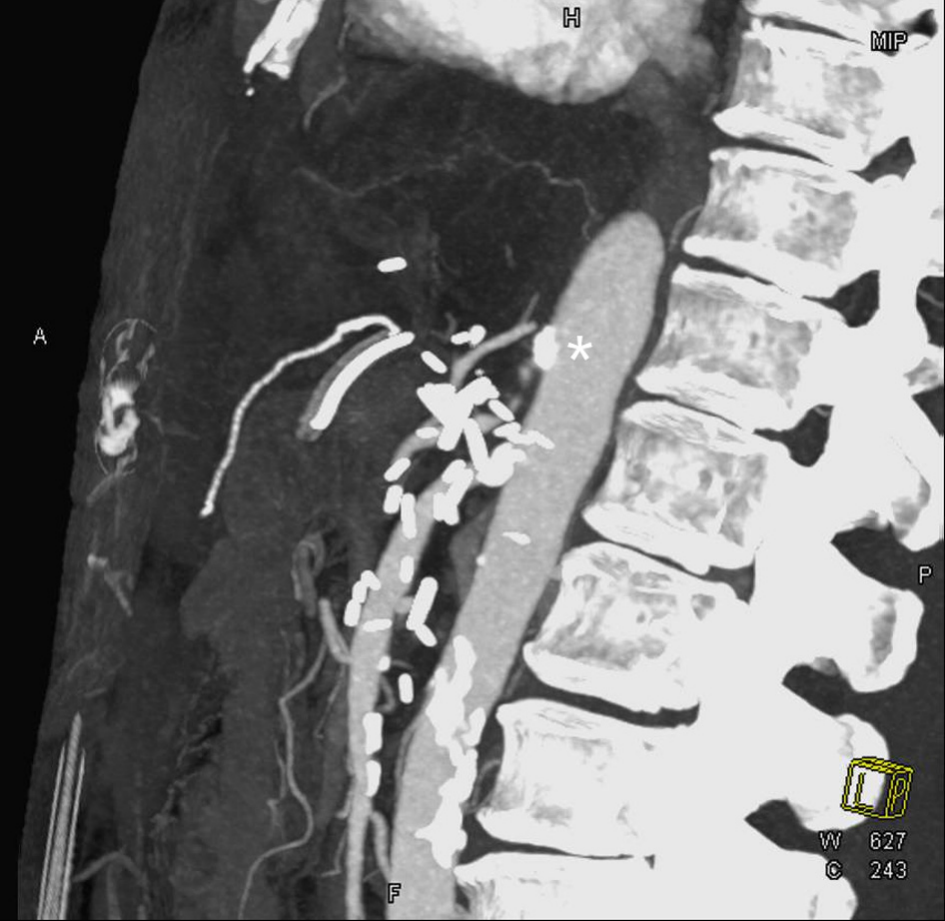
Sagittal image of computed tomography angiography (CTA) of the abdomen and pelvis demonstrating occlusion of the celiac artery (*asterisk*) and a patent superior mesenteric artery (SMA).
